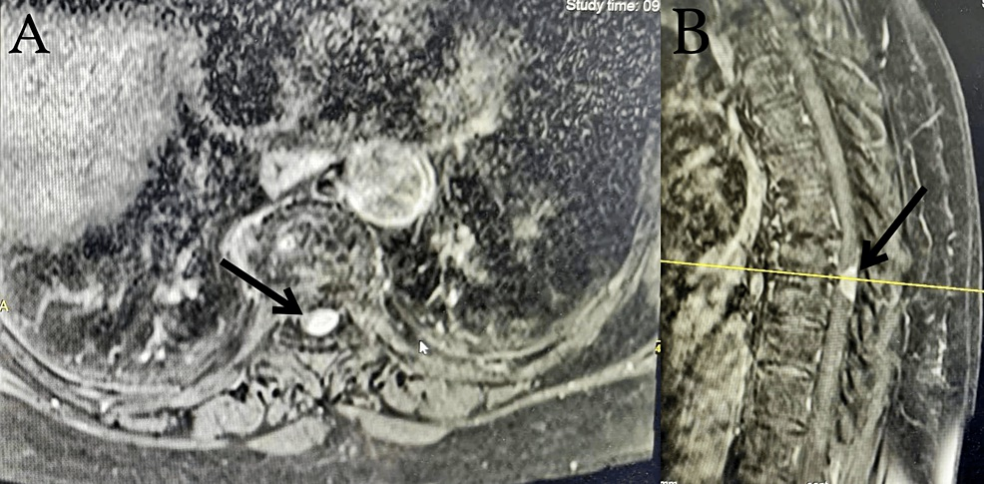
Preoperative MRI of the thoracic spine. A lobulated elliptical mass is seen in the T8-T9 dorsal space (black arrows). (A) Thoracic spine T1-weighted MRI transverse view. (B) Thoracic spine T1-weighted MRI sagittal view. MRI, magnetic resonance imaging.
